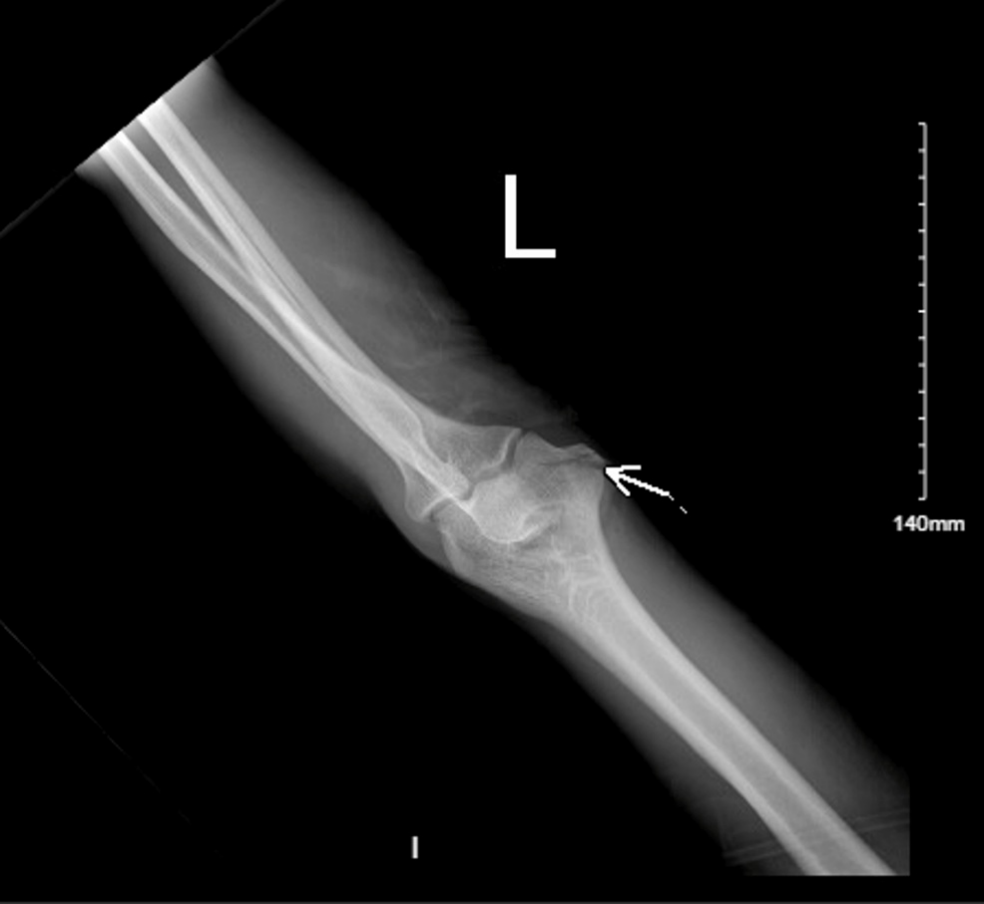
Initial X-ray of the left elbow with medial epicondyle fracture (white arrow)
